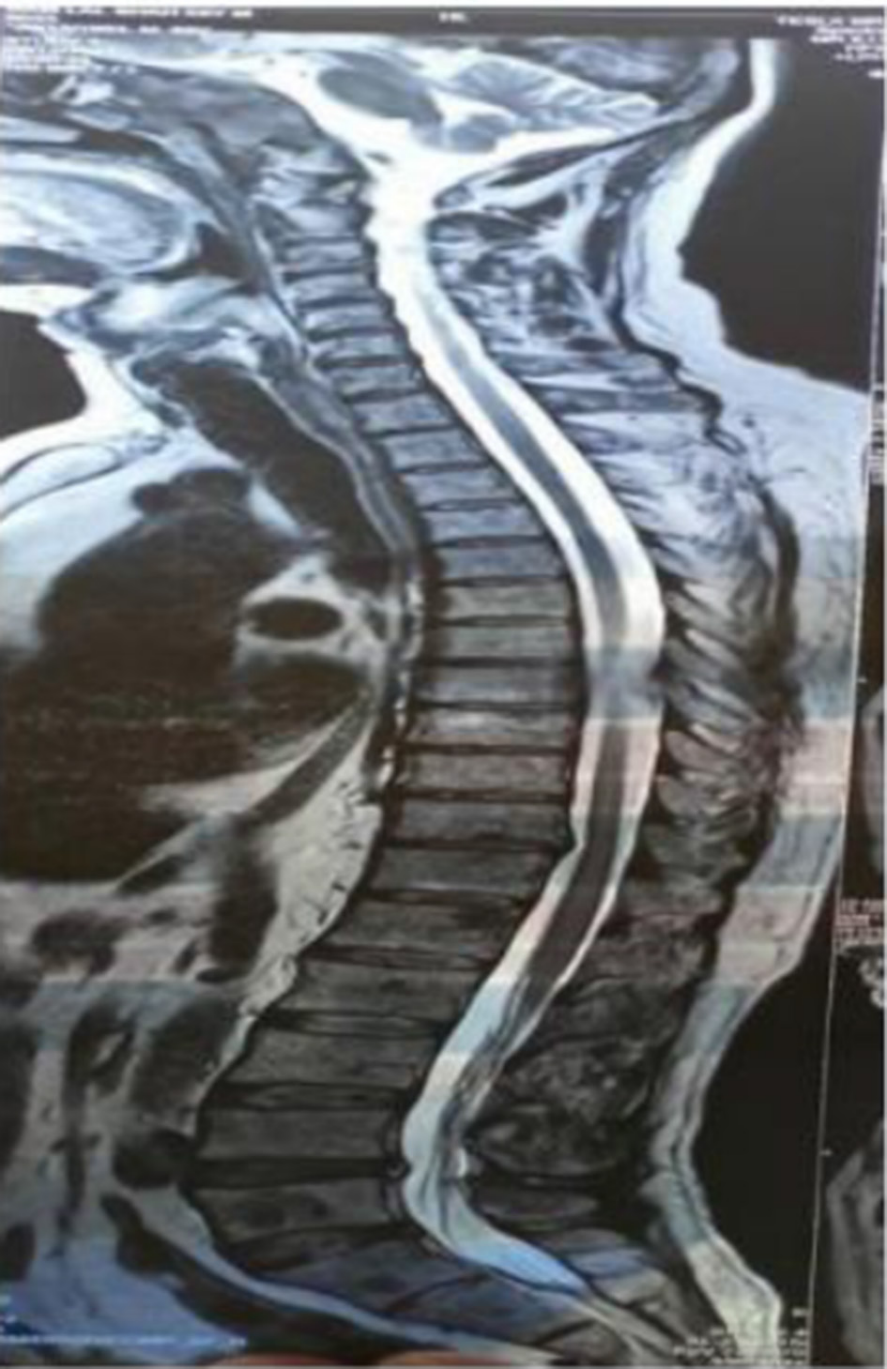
MRI of the whole spine revealed high signal intensity mass replacing the clivus
